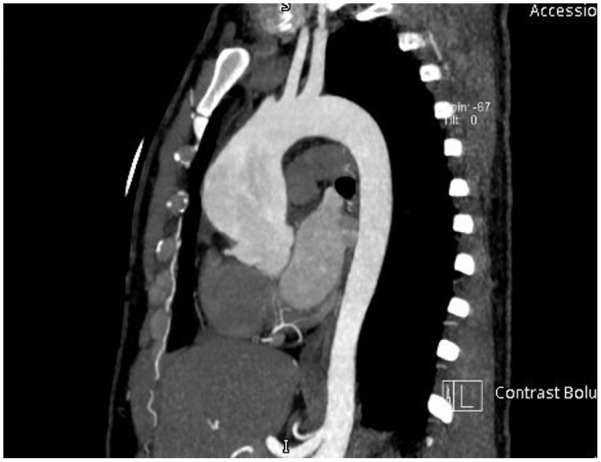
CT angiography of the thoracic aorta showing an ascending aortic aneurysm (maximum diameter approximately 5.0 cm) extending from the aortic root to the proximal arch.
